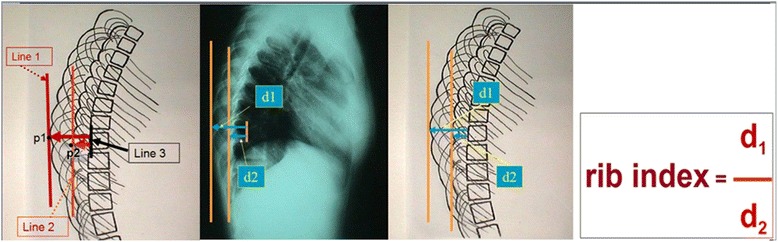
The Rib Index (RI) of the thoracic cage in a standing spinal radiograph.
